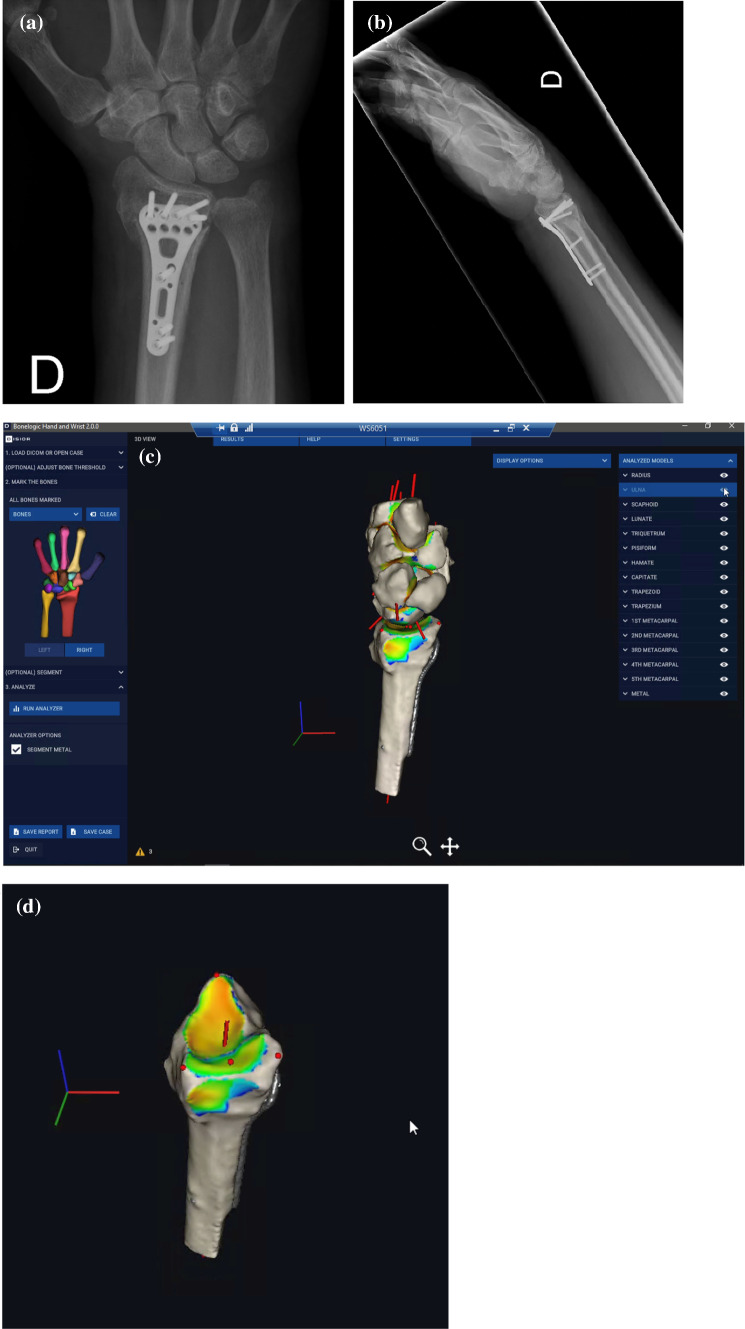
a, b Dorsopalmar and lateral projection of radiographs of a 72-year old male with malunion of a distal radius fracture after open reduction and internal fixation with a volar plate. c Automated three-dimensional assessment of the distal radius malunion with distance mapping of radiocarpal and intercarpal relationships. d Automated three-dimensional assessment of the distal radius malunion with colour mapping showing increased load on the dorsal aspect of the articular surface
